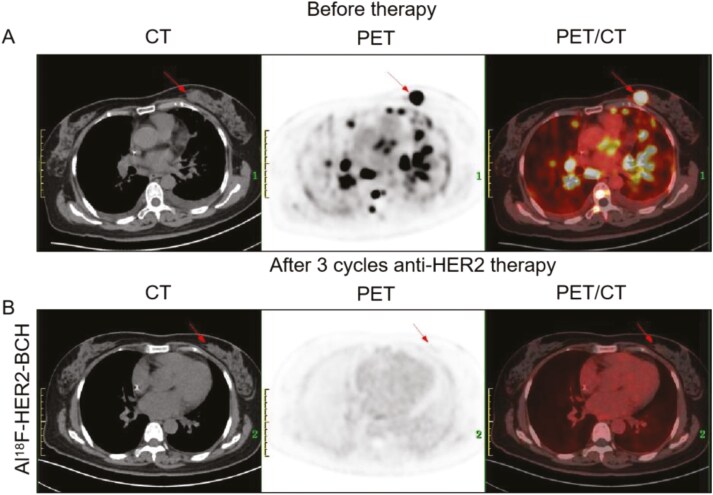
^18^F-FDG (A) and Al^18^F-NOTA-HER2-BCH (B) PET/CT imaging of the same patient with multiple metastases before and after 3 months anti-HER2 treatment. The arrows show lesions.
